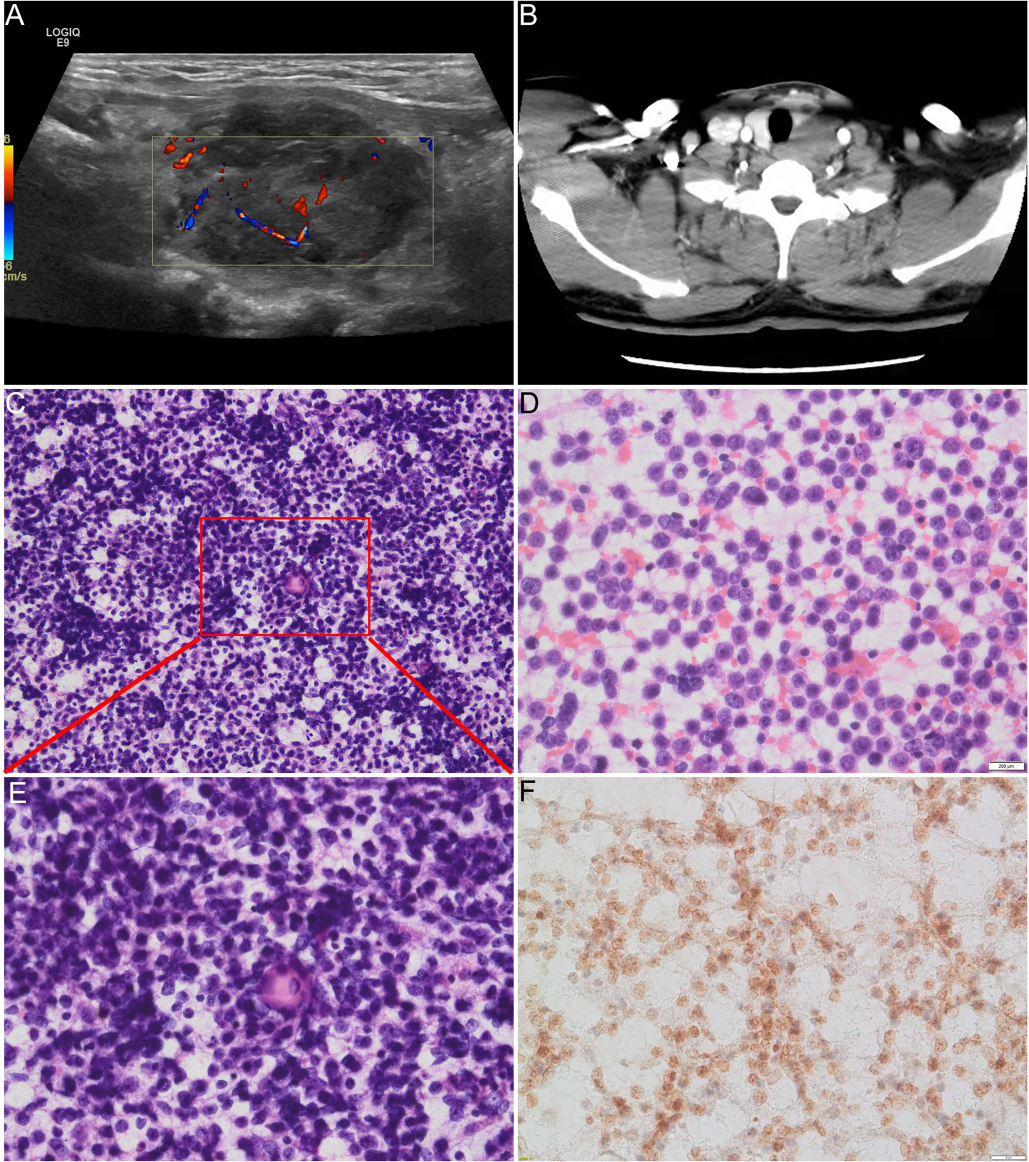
(A) Ultrasound examination revealed a heterogeneous nodule in the left lobe of the thyroid. (B) CT examination revealed 4-cm low-density lesions in the left lobe of the thyroid gland. (C, D, E) Direct cell smears show abundant cells with diffuse distribution of small- to medium-sized atypical monolayer protocells, and the foci of sudden keratosis could be seen from low magnification in (C) and high magnification in (E). (E) Tumor cells have round to oval nuclei with bare and scanty cytoplasm and vague to prominent nucleoli. The chromatin of the nucleus varies from mostly light open chromatin to hyperchromatic. (F) Positive immunoreaction with NUT protein in the nucleus of the carcinoma cells.
